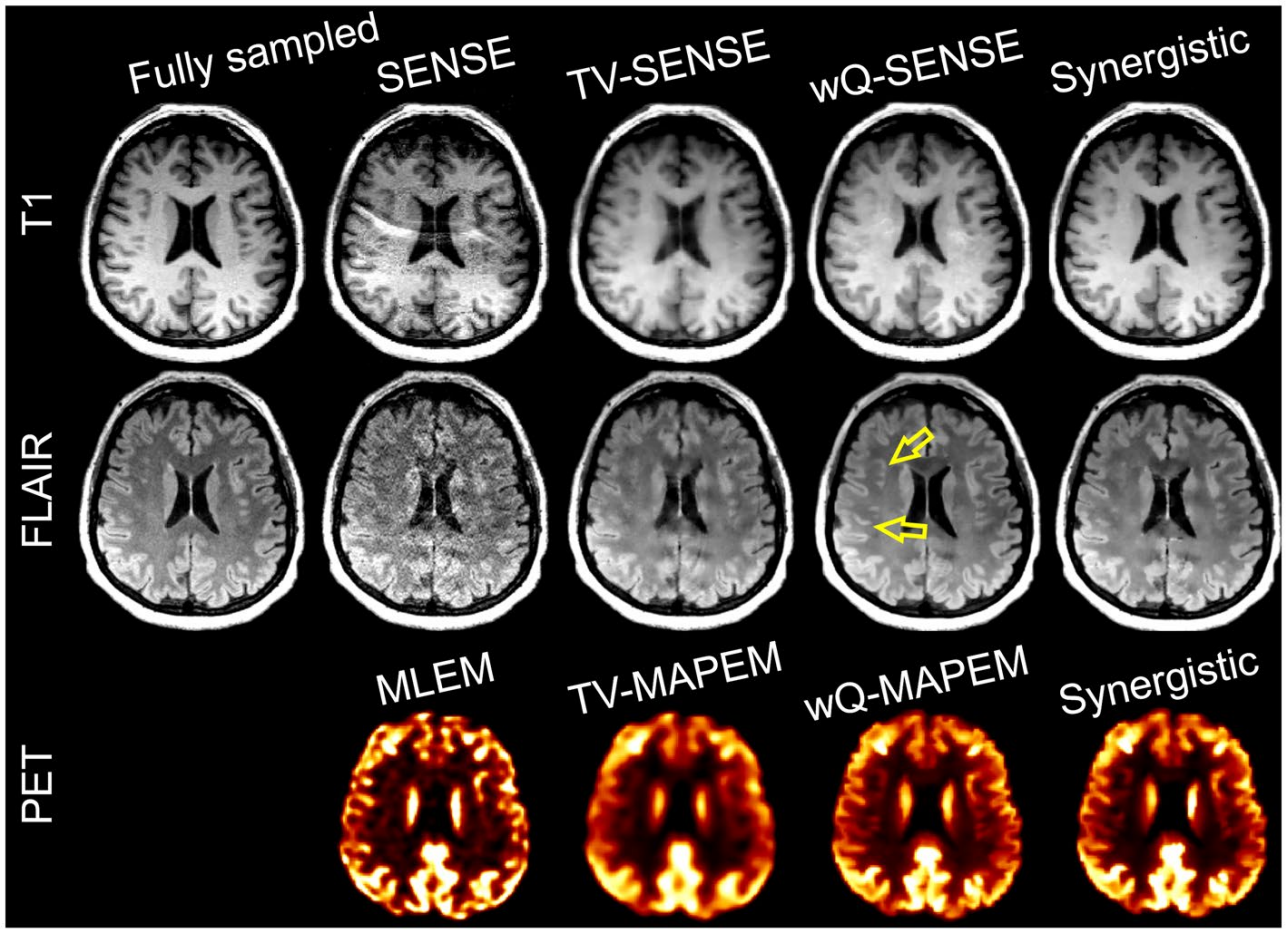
Synergistic PET‐MR image reconstruction of the PET‐MR dataset in comparison with the conventional and separate reconstruction methods.
